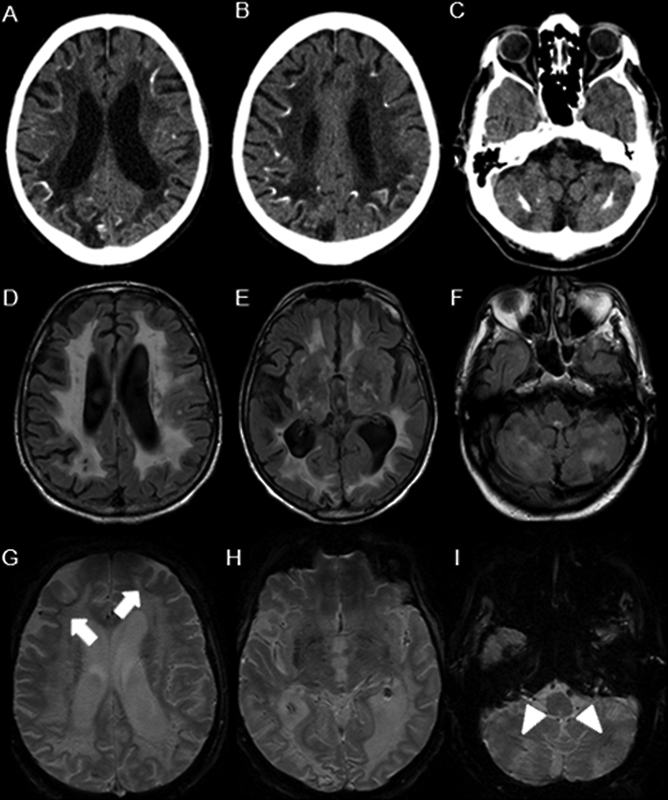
Axial non-contrast computed tomography (CT) scan of the brain showing diffuse hypodensity in the deep white matter of both cerebral hemispheres with cerebellar and cerebral subcortical calcifications (A-C). Axial T2/fluid-attenuated inversion recovery (FLAIR) magnetic resonance imaging (MRI) scan showing confluent hypersignal in the periventricular, subcortical, and deep white matter of both cerebral hemispheres (D), the thalamus (E), and the cerebellar hemispheres (F), with hypointense areas involving the basal ganglia (E). Axial gradient echo (GRE) T2-weighted magnetic resonance imaging (MRI) scan showing subcortical white matter (G: arrows) with either microbleeds or mineralization in the basal ganglia (H) and cerebellar hemispheres (I: arrowheads).
